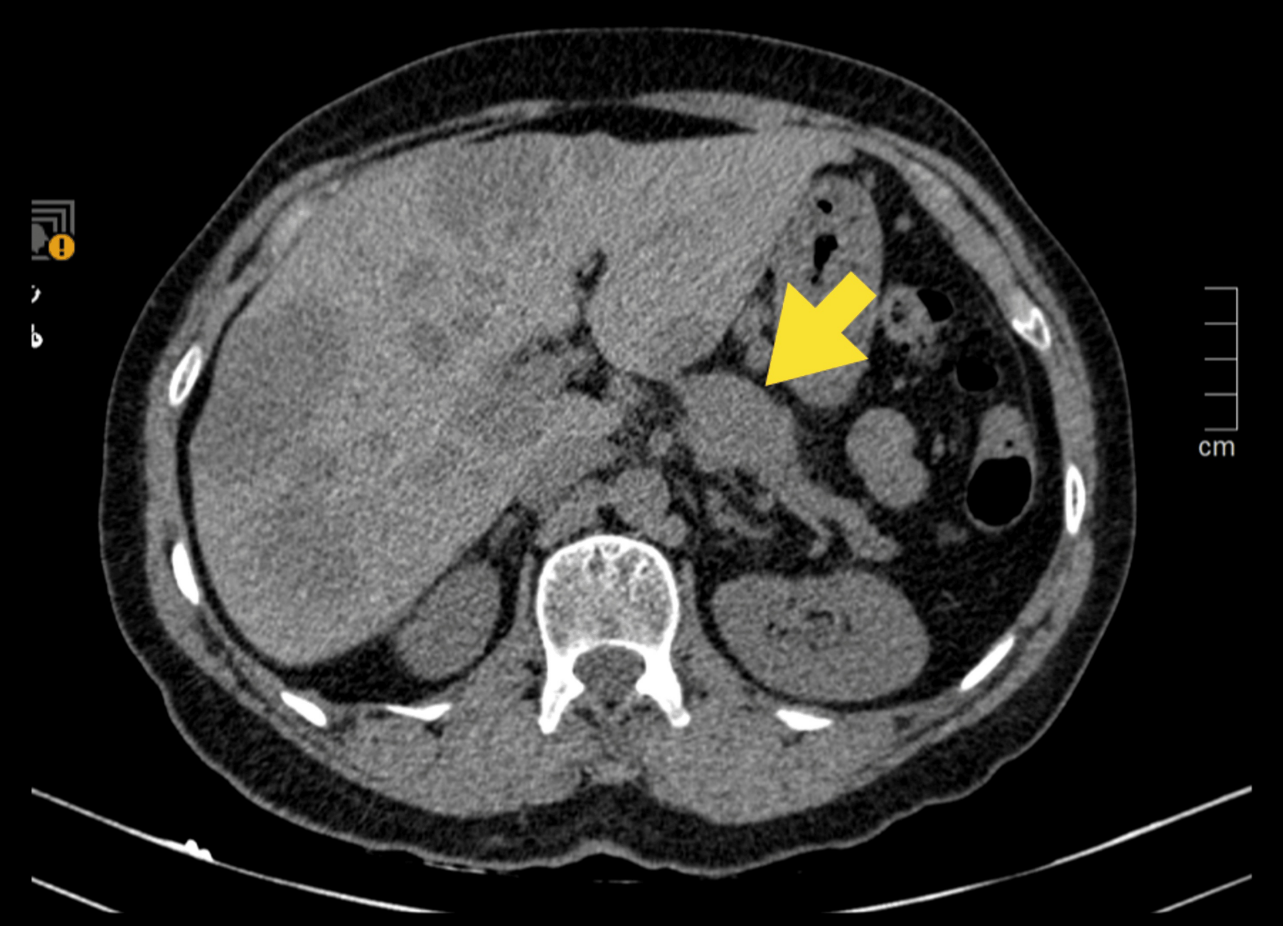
Axial abdominal-pelvic CT scan Liver with lobulated contours and multiple hypodense nodular lesions and a hypodense area in the body region of the pancreas (yellow arrow) CT: computed tomography
